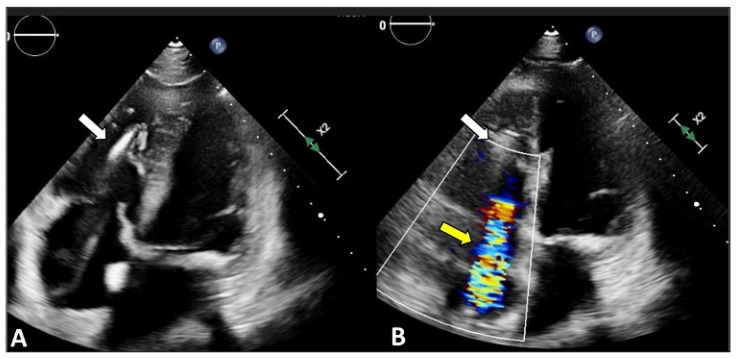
Cardiac implantable electronic device (CIED)-related tricuspid regurgitation (TR). Transthoracic echocardiogram, apical four-chamber view documents CIED RV lead (A,B, white arrows) and RA lead that cause relevant TR visible with Color-doppler (B, yellow arrow).
